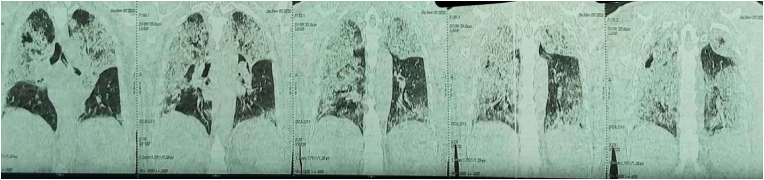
Chest CT scan showing frosted glass images.
